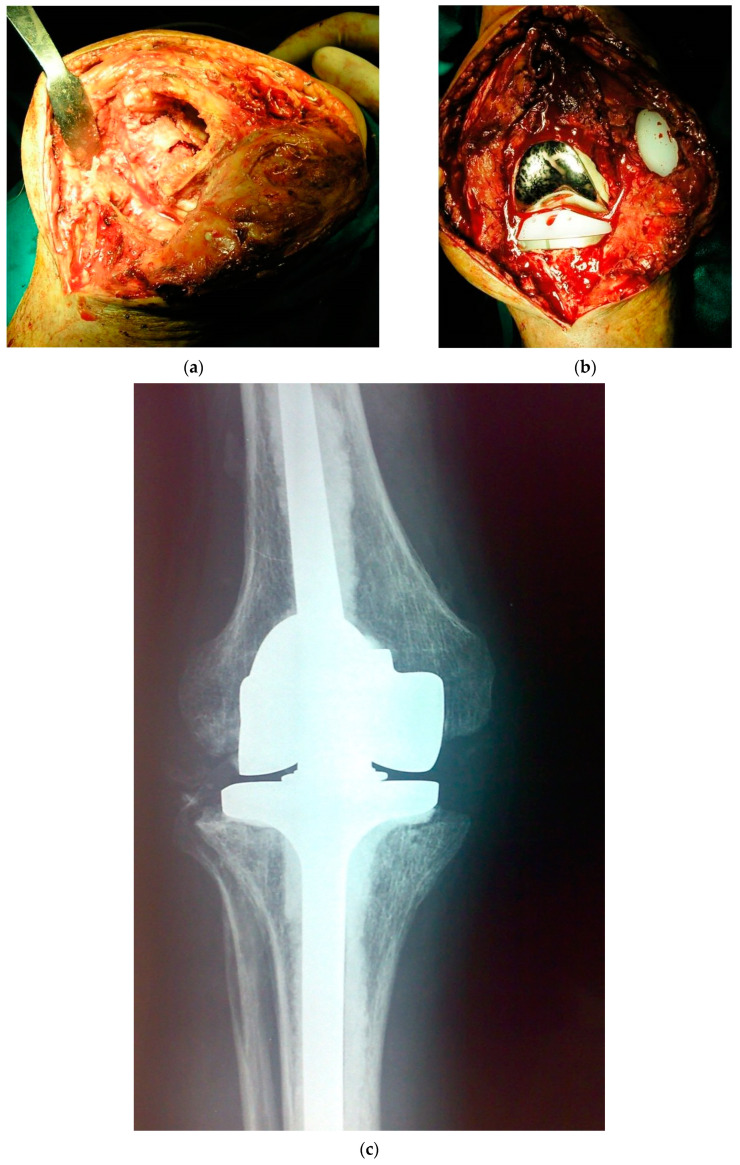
(a–c). Images of the same patient of Figure 1 and Figure 2 relative to the second stage of the two-stage revision arthroplasty: (a) Intraoperative image after removal of the articulated spacer; (b) Intraoperative image with the new prosthesis implanted; (c) Postoperative radiograph of the implanted prosthesis (rotating hinge total knee arthroplasty).
